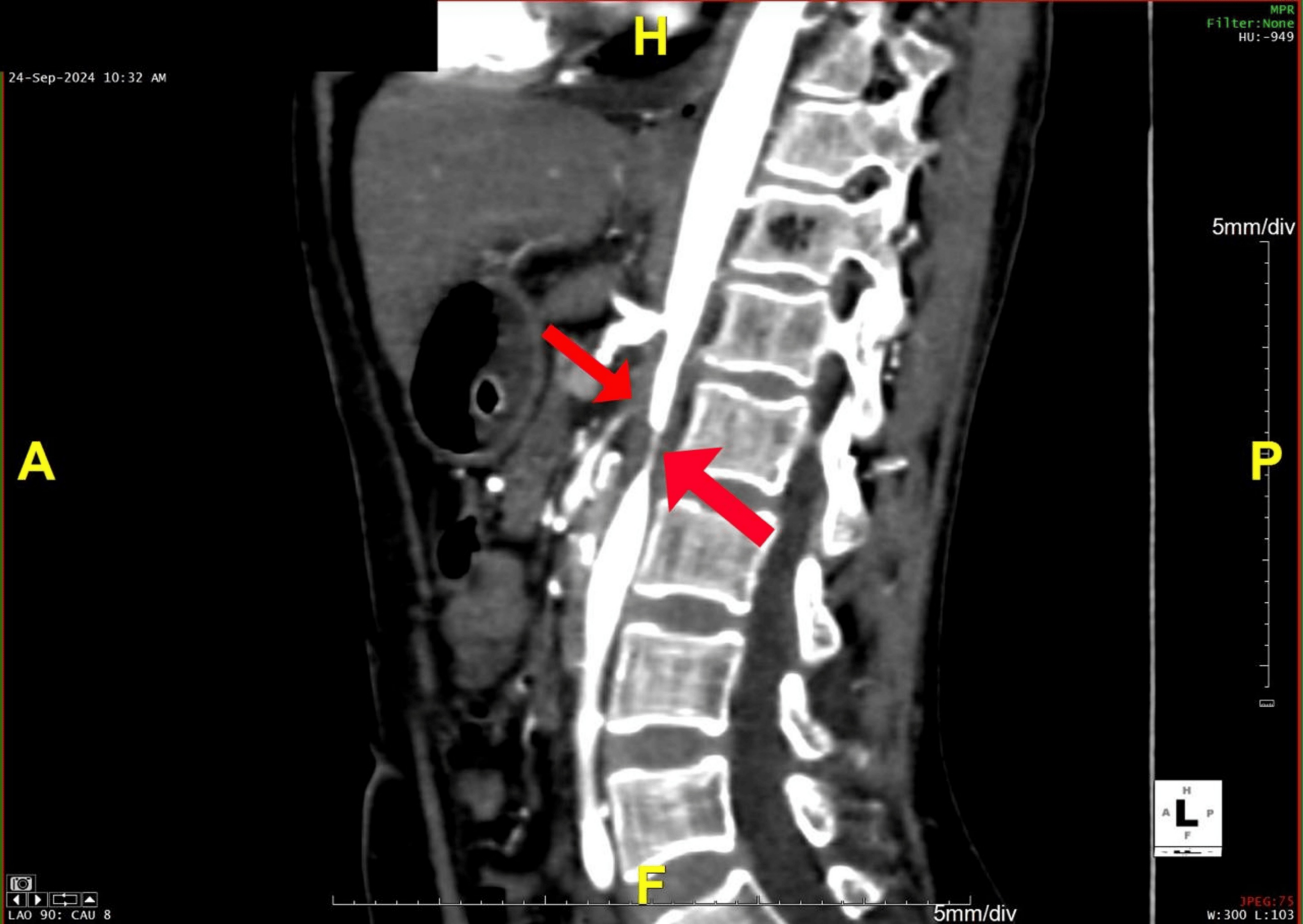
A sagittal section of the CT angiogram reveals narrowing of the abdominal aorta and superior mesenteric artery CT: computed tomography
